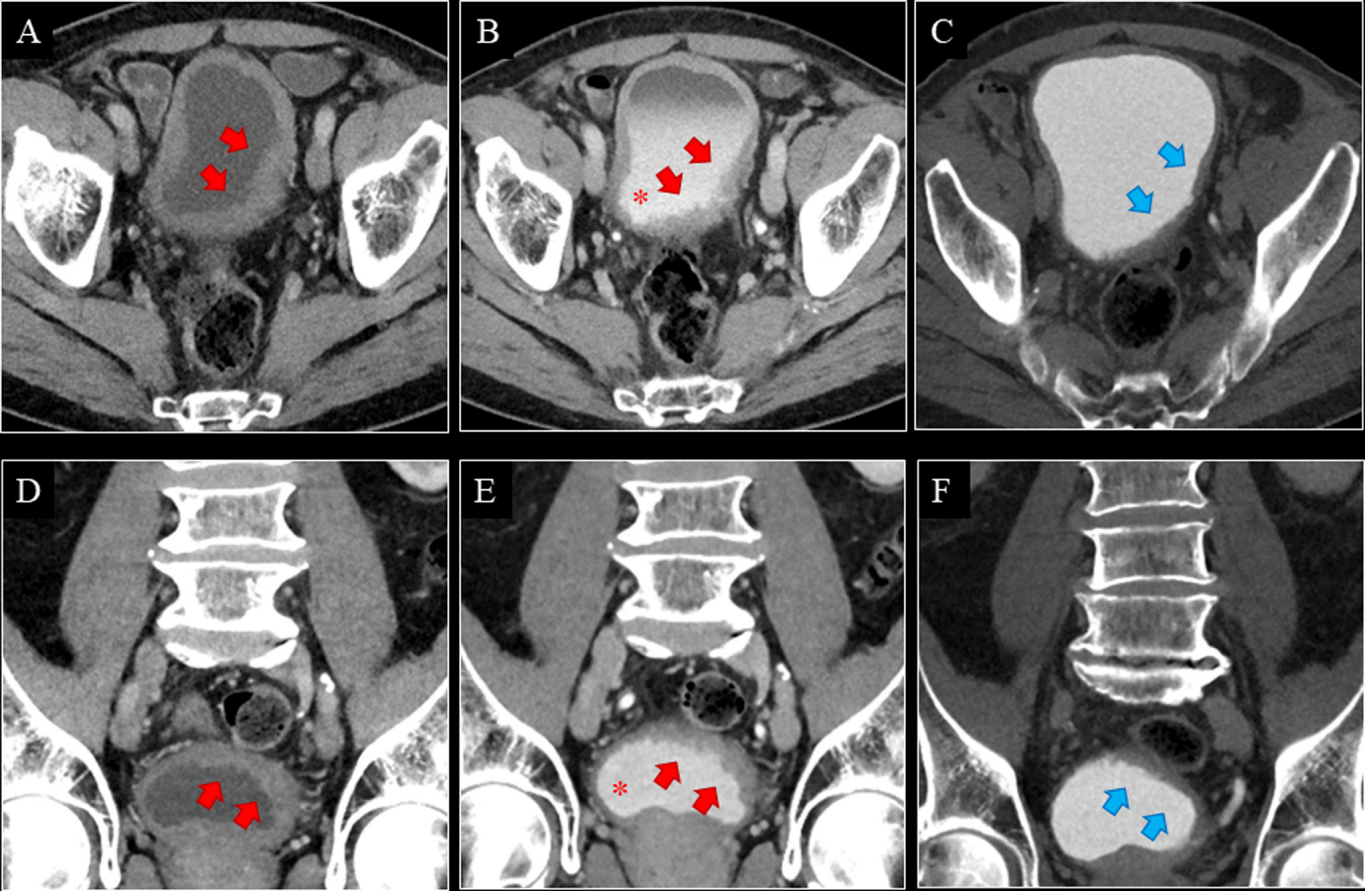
A 76-yr-old male with a flat growing pT1 tumour in the left bladder wall. For the primary reading team, the experimental CT was positive and the control CT was negative for bladder UC. For the secondary reading team, both the experimental and the control CT were negative for bladder UC. Retrospectively, the tumour (red arrow) was visible on (A and D) CMP, (B and E) NP, and (C and F) possibly EP (blue arrows).The contrast medium was accidentally injected subcutaneously. Consequently, the automatic bolus tracking was unsuccessful, and the CMP was started manually after 45 s. Then, the patient received a second contrast injection, which was successfully injected intravenously, and the NP was performed at 70 s after the threshold of the second contrast injection, 7 min after the first contrast injection. This is why secreted contrast was seen in the urine on the NP (red asterisk). A poorly filled bladder on CMP and NP, and a smoothed-out bladder wall on EP were interpreted as bladder hypertrophy only by some of the readers. CMP = corticomedullary phase; CT = computed tomography; EP = excretory phase; NP = nephrographic phase; pT = pathological tumour stage; UC = urothelial carcinoma.
